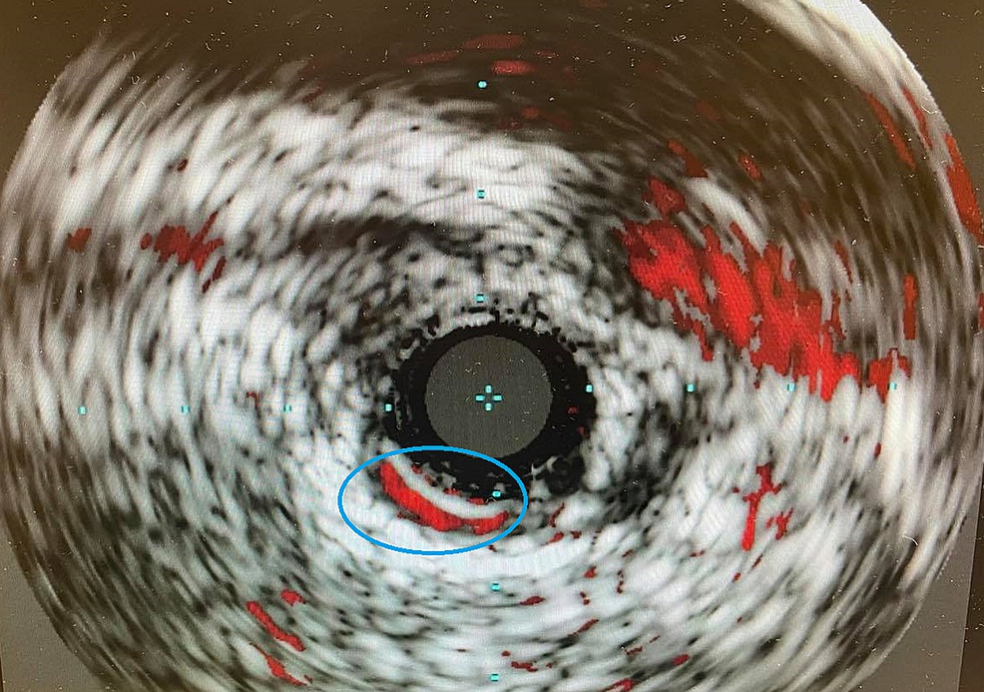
IVUS shows type A spontaneous coronary dissection. IVUS, intravascular ultrasound
